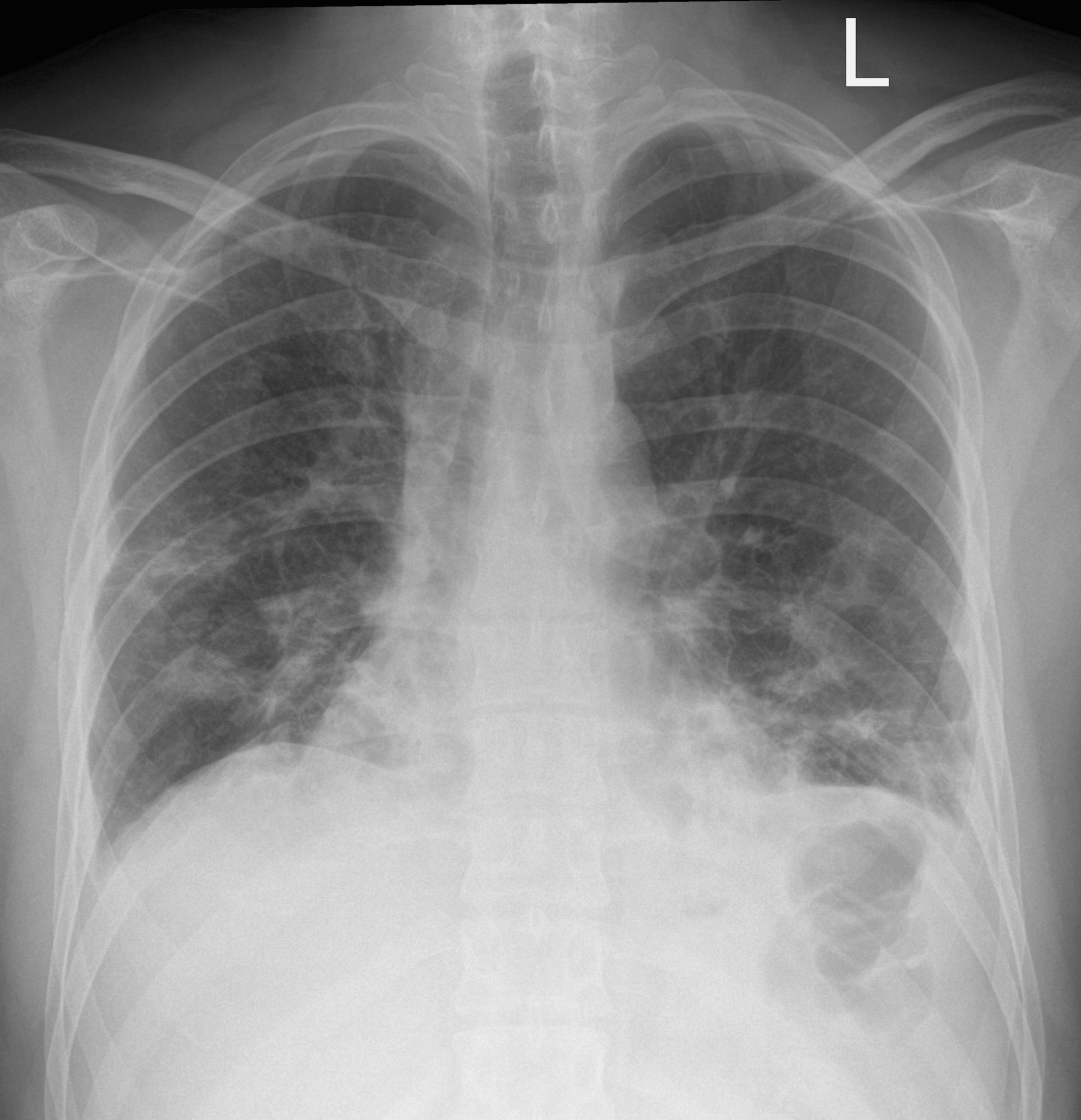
Chest X-ray showing bilateral infiltrative lung changes
